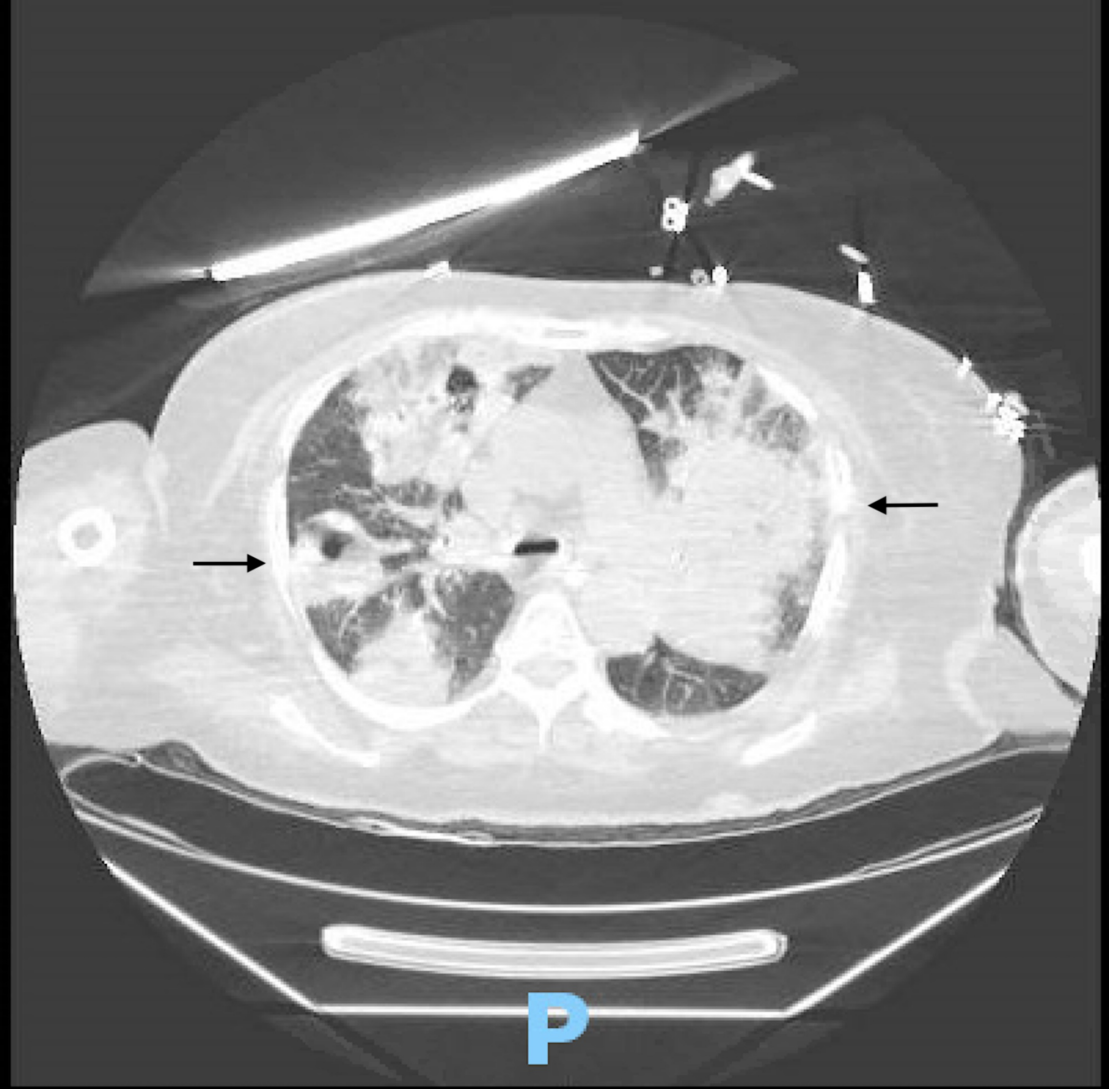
Chest computed tomography with intravenous contrast showed multifocal consolidations with central ground-glass opacities and cavitation, concerning for angioinvasive aspergillosis
